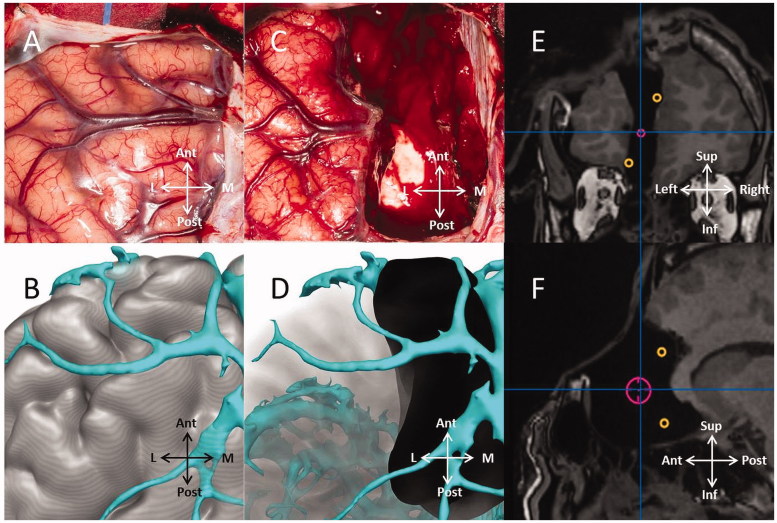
Pre- and post-operative recording of left frontal resection. (A) Intra-operative photograph of left frontal region. (B) EpiNav^TM^ modelling of gyral and vascular anatomy. (C) Intra-operative photograph of left frontal resection. (D) EpiNav^TM^ reconstruction of completed resection. (E and F) Coronal and sagittal intra-operative MRI showing completed resection, incorporating neurophysiological landmarks of ictal onset (red) and propagation to anterior cingulum and medial orbitofrontal area (orange.) (Ant: anterior; Post: posterior; L: lateral; M: medial; Sup: superior; Inf: inferior) (see online version for colour figures).
